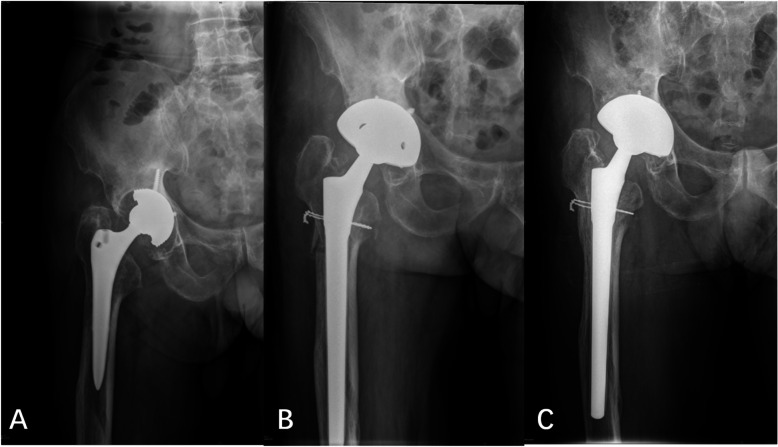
Preoperative and postoperative radiographs of tapered stem with intraoperative fractures
